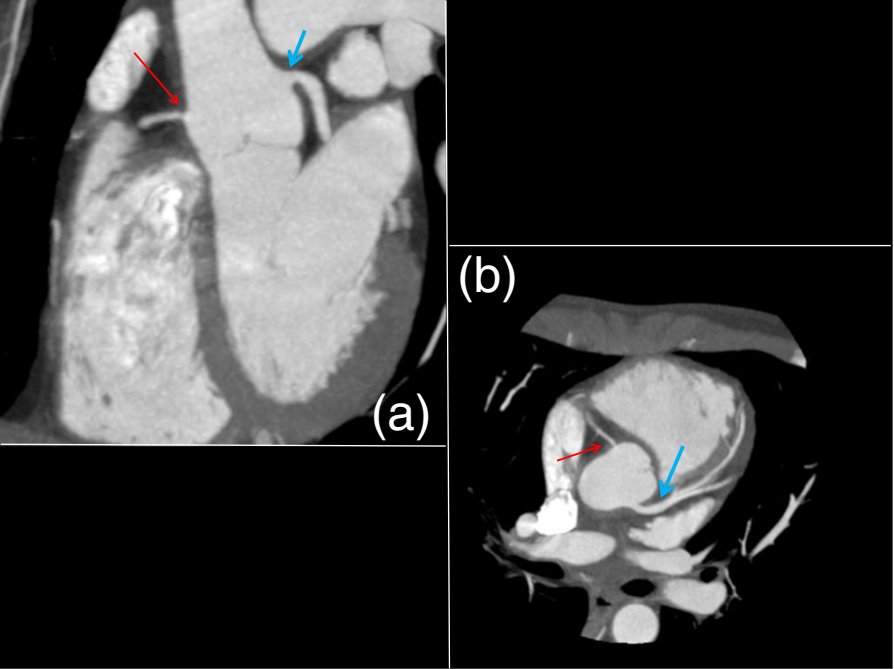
Coronal (a) and Axial (b) Cardiac Gated CT Chest 4 months after systemic therapy. The right coronary artery, though still stenotic, was improved and now able to be visualized on coronal section (a, thin red arrow). The left coronary artery opened up significantly (b, blue arrow).
